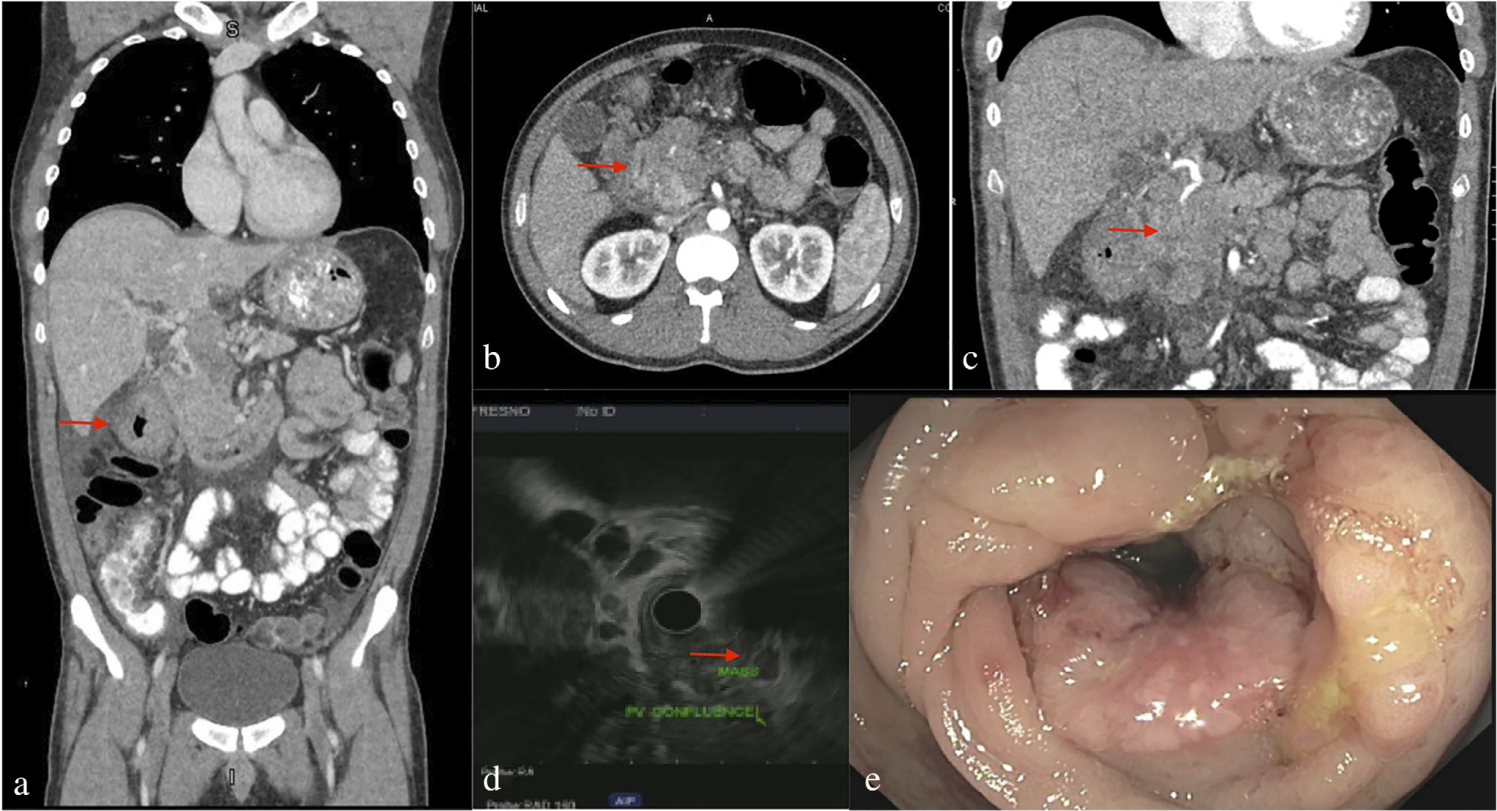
(a) Computed tomography (CT) scan showing large apple‐core, non‐obstructive mass in proximal transverse colon. (b, c) CT scan demonstrating pancreatic head mass. (d) Endoscopic ultrasound showed irregular, hypoechoic, heterogeneous lesion with portal vein invasion. Staged T3N2MX. (e) Colonoscopy showing partially obstructing mass with abnormal, ulcerated mucosa in the proximal transverse colon.
